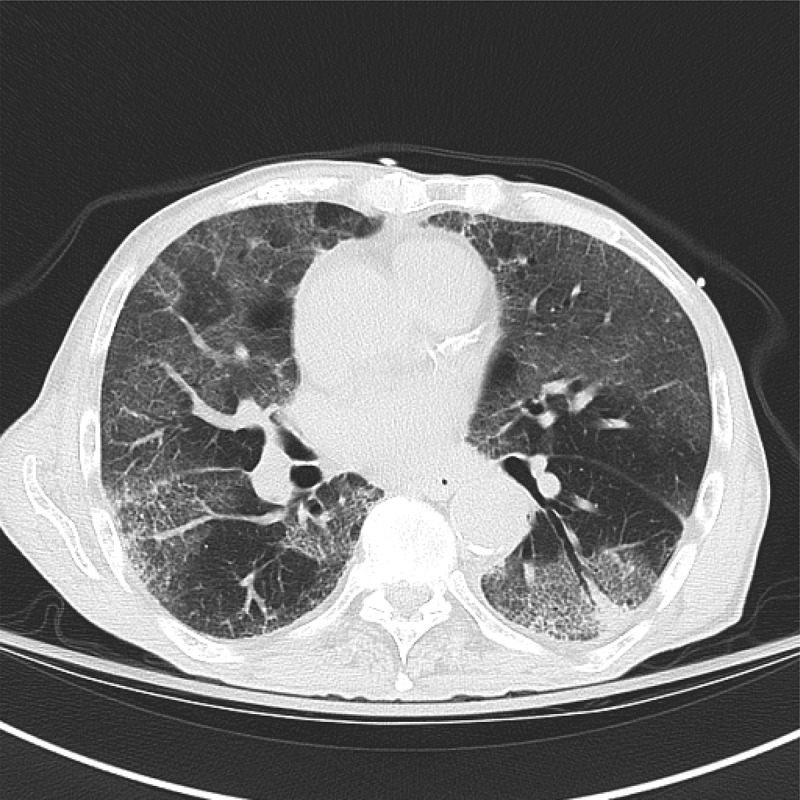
CT scan of chest showing bilateral diffuse ground glass opacity of lung parenchyma on presentation.
